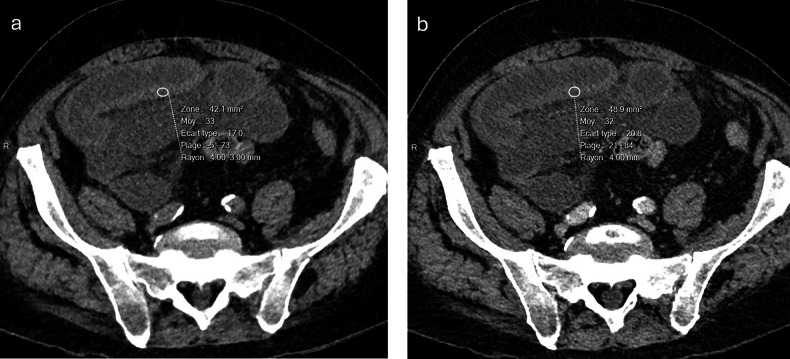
Axial, conventionally acquired, polychromatic non-enhanced (a) and portovenous (b) multi-detector CT images demonstrate acute bowel ischaemia resulting from acute small bowel obstruction in an 88-year-old male patient. Quantitative analysis of the bowel wall revealed no enhancement during the portovenous phase (b), with a mean density of 33 Hounsfield units (HU) in a and 32 HU in b
